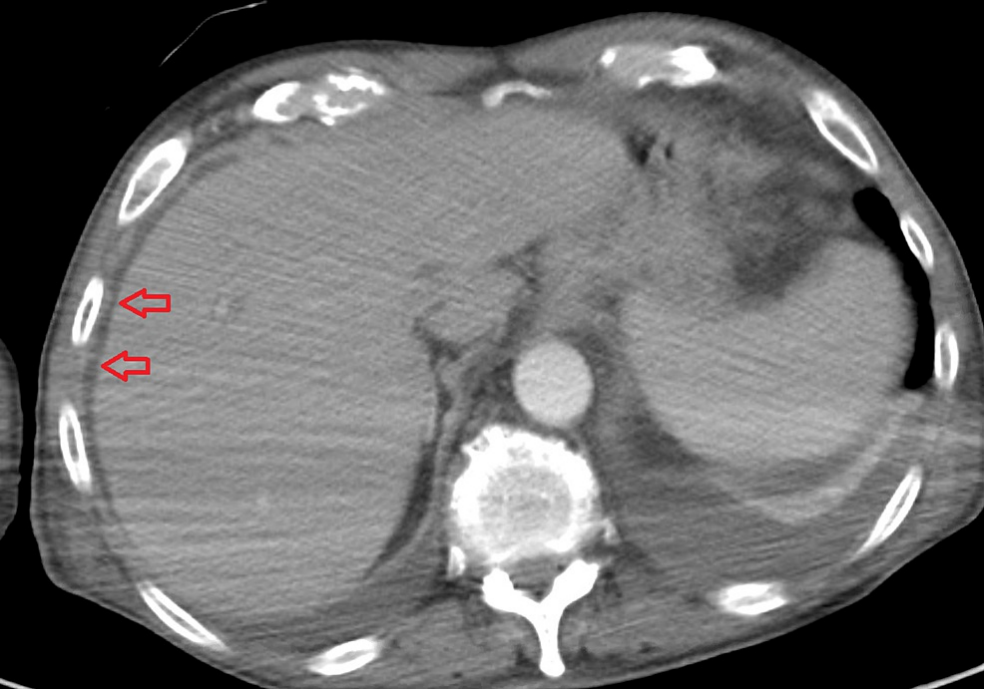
Computed tomography (CT) scan of the abdomen on admission. Red arrows: Area of mild perihepatic fluid collection noted.
